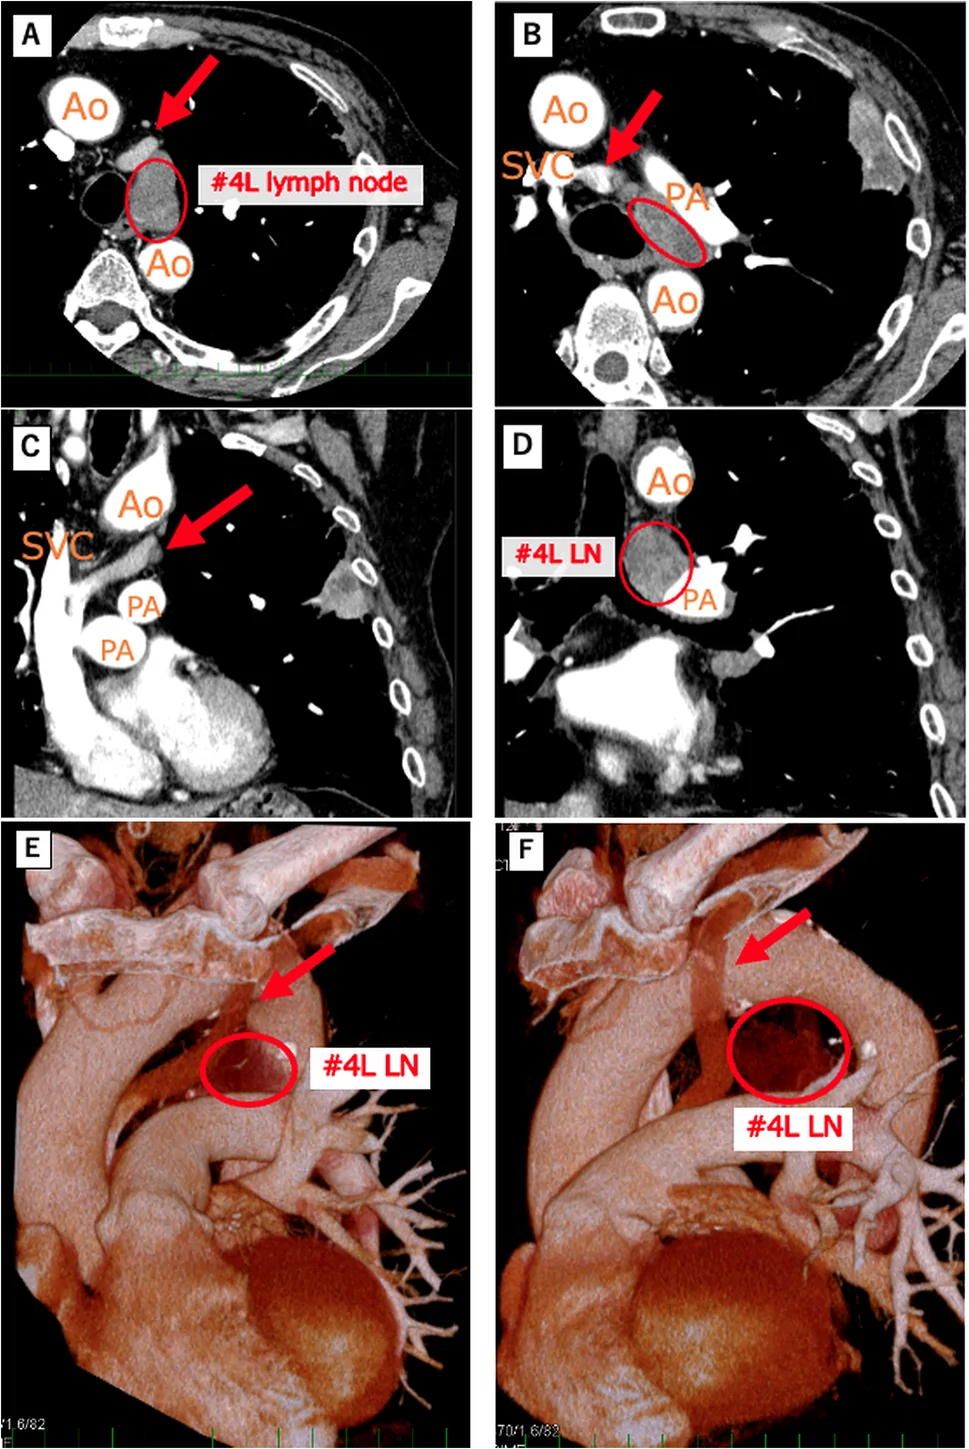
Preoperative contrast-enhanced computed tomography (CT) and three-dimensional (3D) reconstruction. (A, B) Axial CT images and (C, D) coronal CT images demonstrate a subaortic anomalous left brachiocephalic vein coursing through the aortopulmonary window, in relation to the enlarged station 4 L lymph node and left pulmonary artery. (E, F) Enlarged three-dimensional reconstruction images show the anomalous left brachiocephalic vein crossing the aortopulmonary window and the station 4 L lymph node. The anomalous left brachiocephalic vein is indicated by arrows, and the station 4 L lymph node is highlighted by an oval. Ao, aorta; PA, pulmonary artery; SVC, superior vena cava
